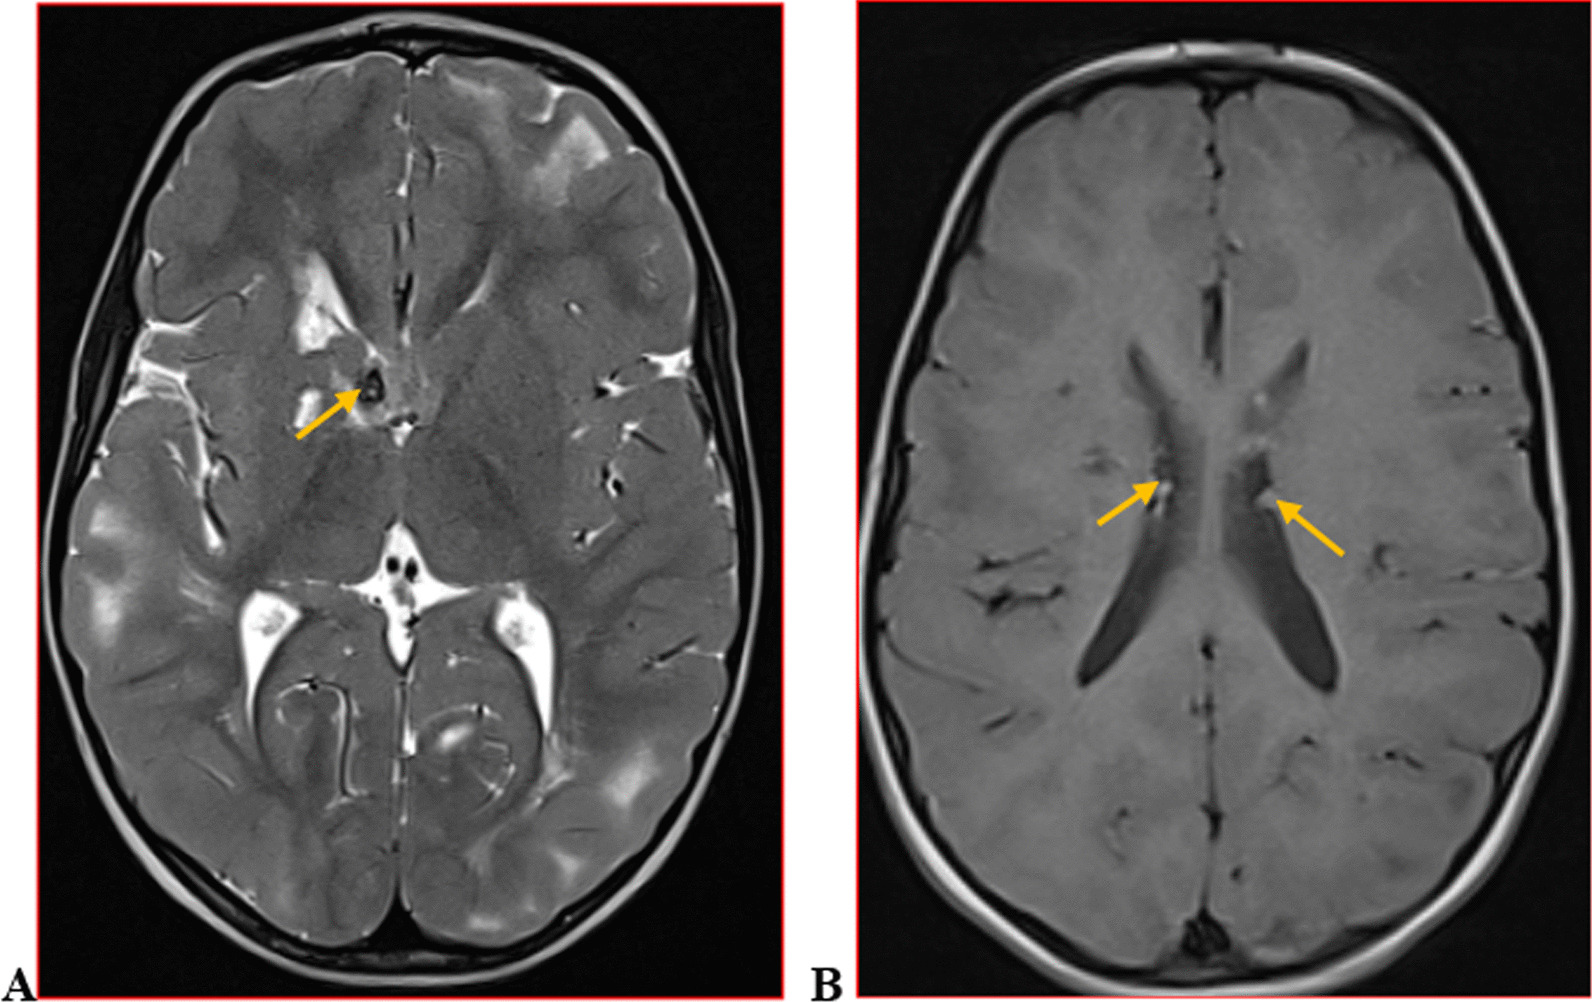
Tuberous Sclerosis Complex in a three year old male with epileptic spasms. Axial T2-weighted (A) brain MRI showing cortical/subcortical tubers as well-circumscribed areas of high signal intensity in the left frontal and occipital lobes and in the right temporal and occipital lobes representing subcortical tubers; T2-hypointense nodule in right foramen of Monroe representing a Subependymal Giant cell Astroytoma (SEGA) (arrow). Axial T1-weighted MRI (B) showing isointense nodules arising from the walls of the lateral ventricles (arrows) representing subependymal harmatomas
